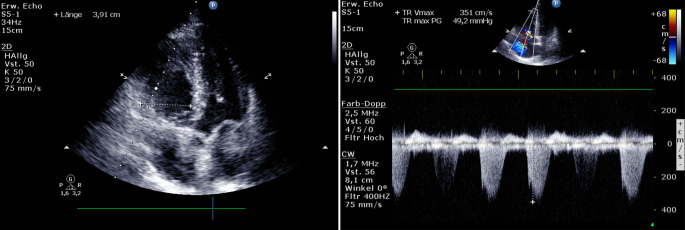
Case—echocardiography [42, 43]
